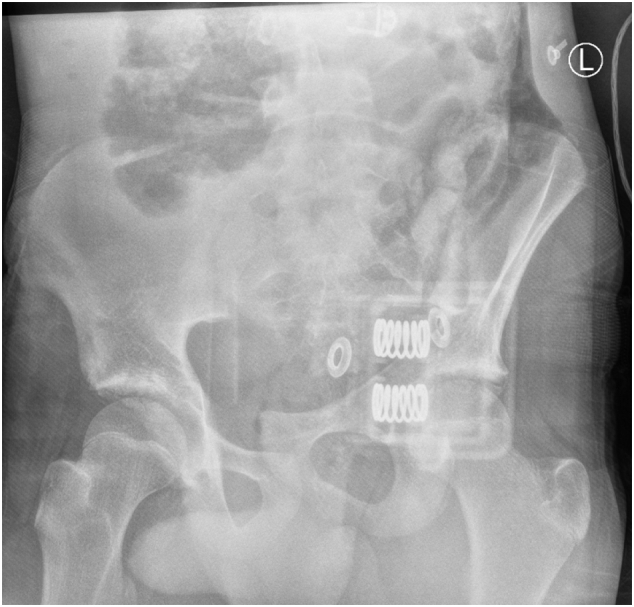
Plain films demonstrated fractures left sacrum and iliac bone and LSP.
